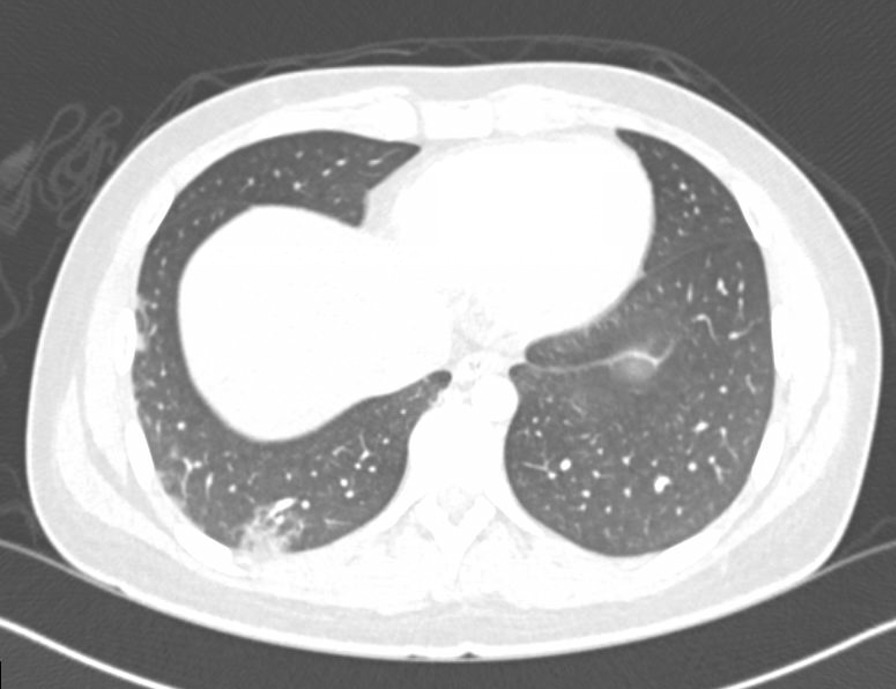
Chest CT imaging showing ground glass opacities
